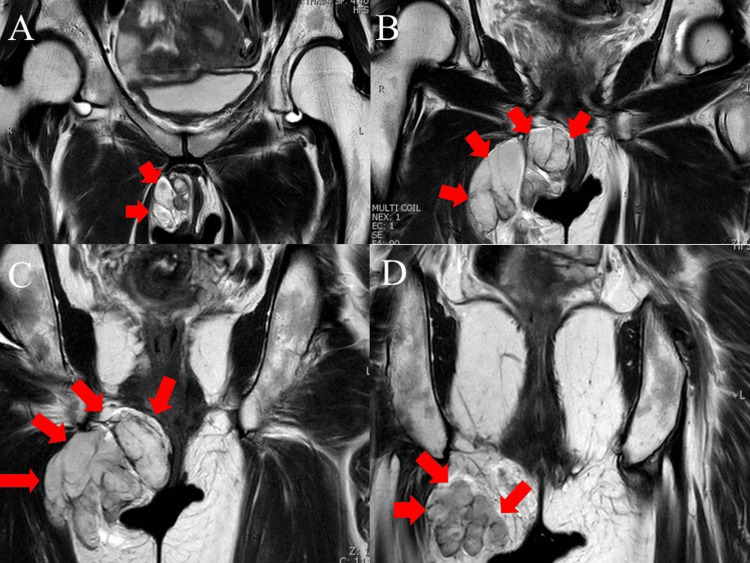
Magnetic resonance images. Coronal section of T2-weighted image. T2-weighted image shows a hyperintense signal. The images from A to D are ventral to dorsal.
